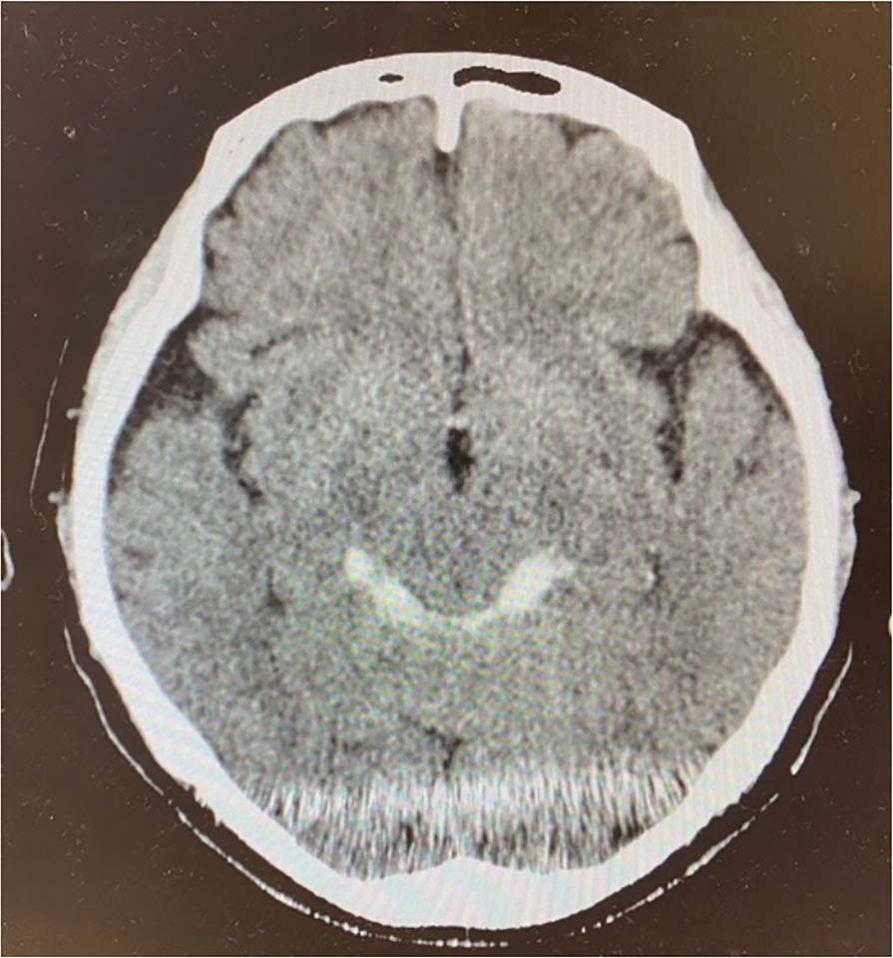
Brain computed tomography on day 3 of the patient’s hospitalization, showing a small high-density area indicating hemorrhage in the bilateral ambient cistern.
